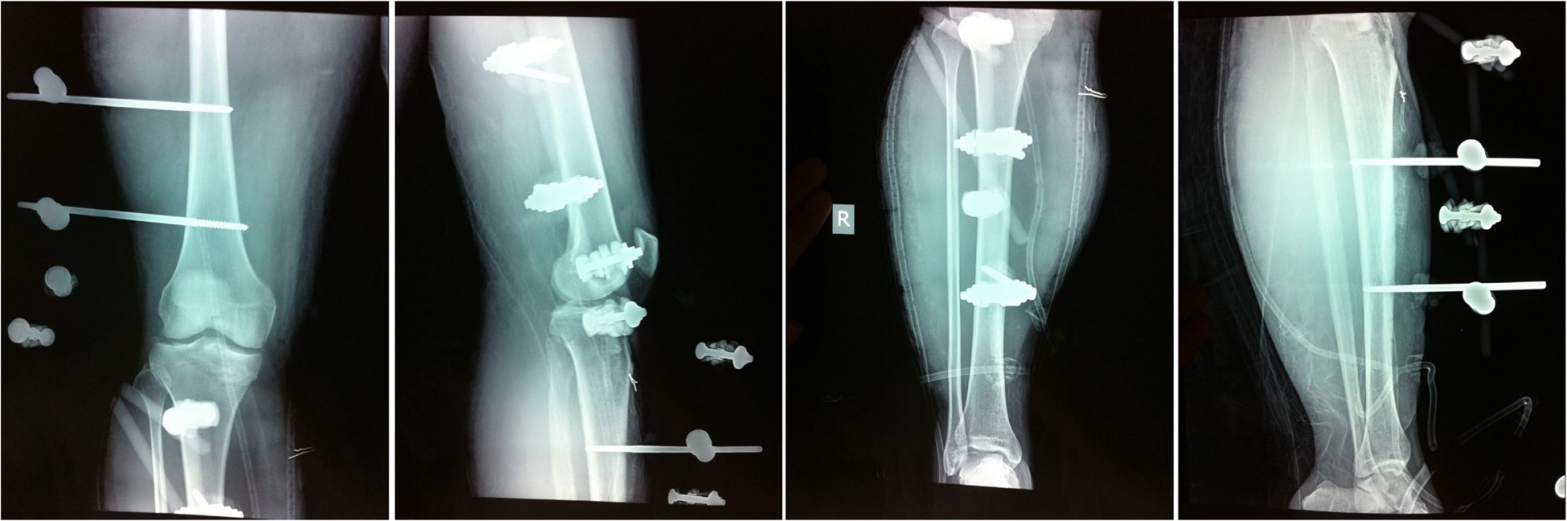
Postoperative radiographs. The fracture was fixed with external fixator
